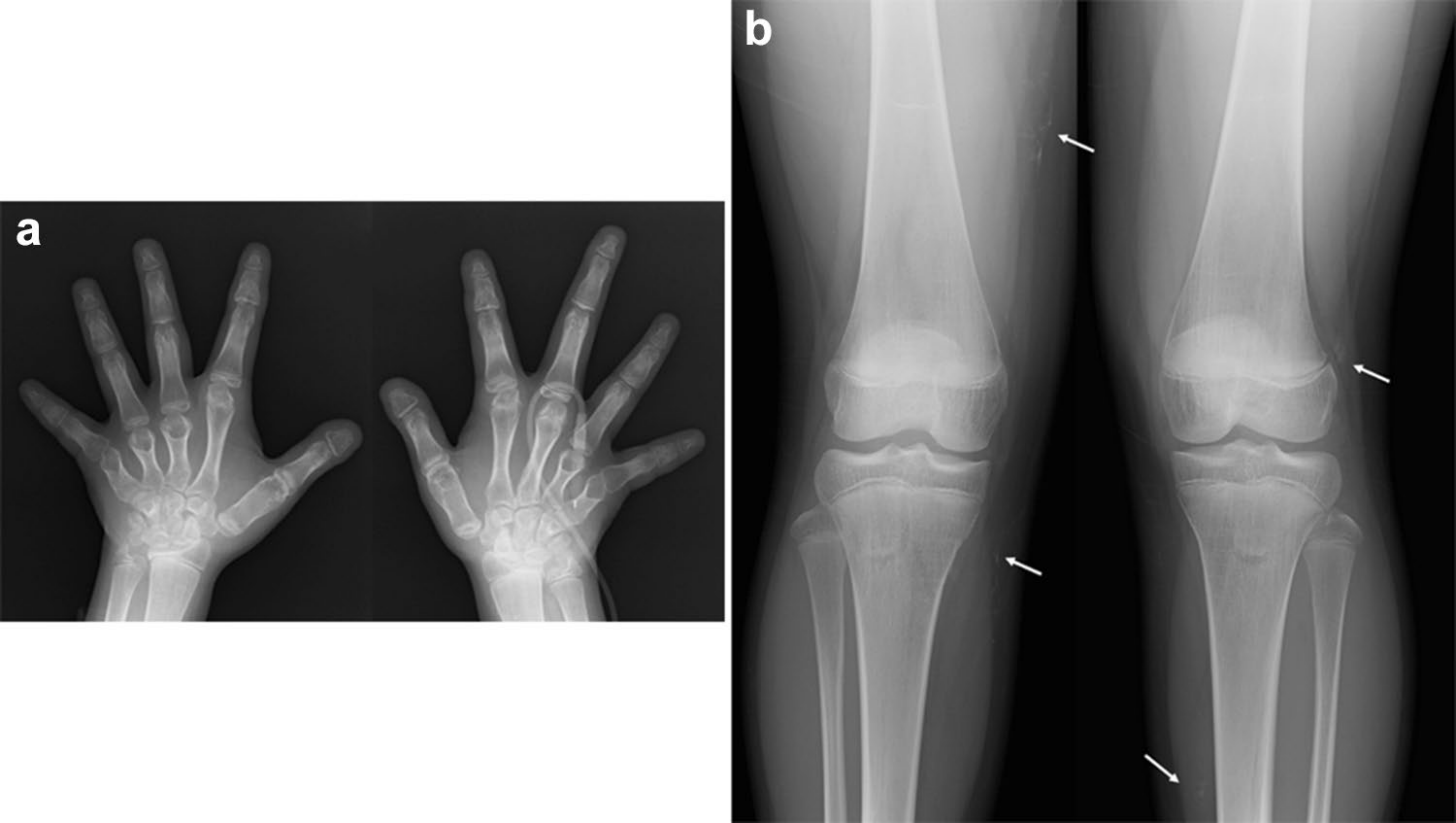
a, b A 12-year-old girl with Albright hereditary osteodystrophy. Radiographs show shortening of the ulnar metacarpals (type E brachydactyly) and short distal phalanges, particularly of the thumb, and periarticular soft tissue calcifications around both knees (arrows)
